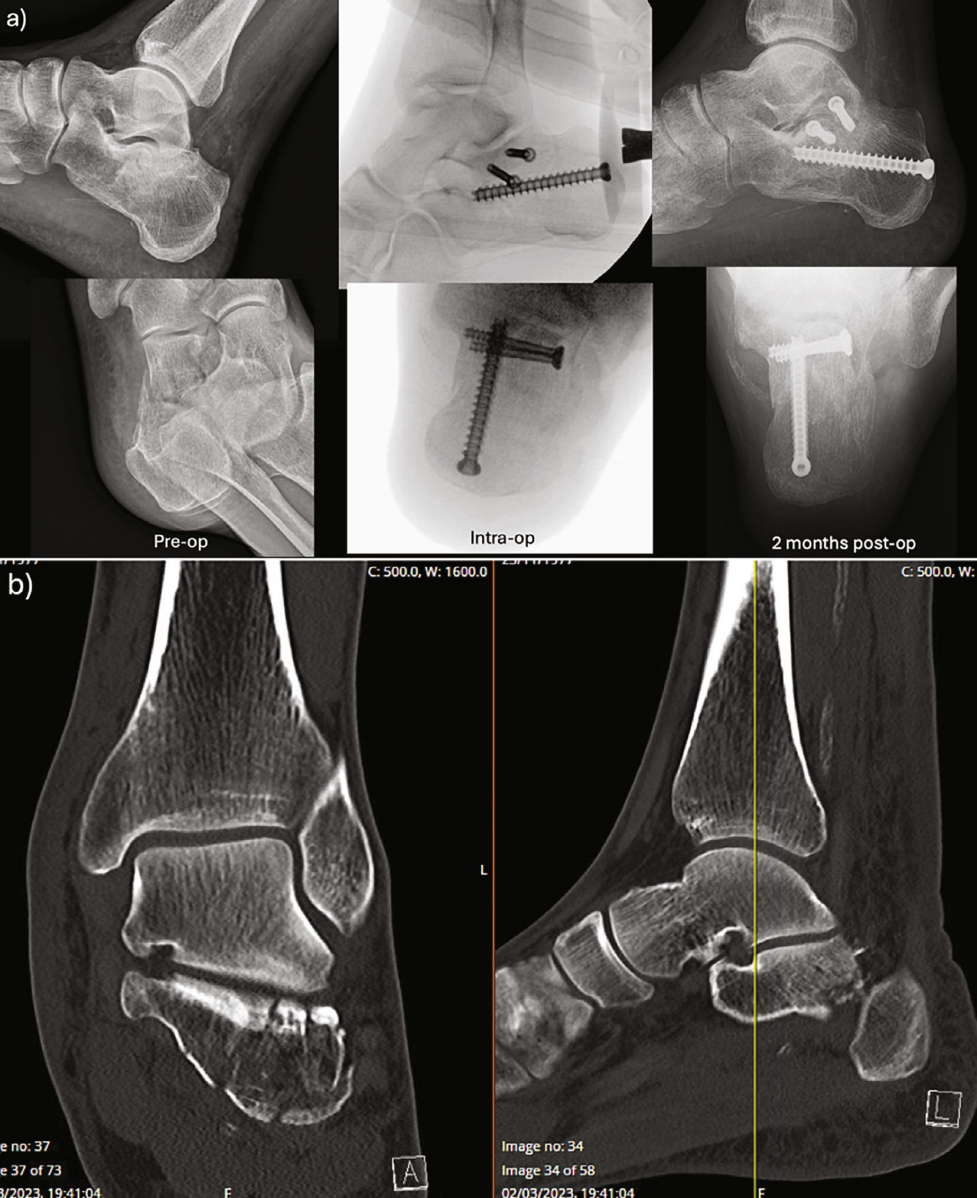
Case B. (a) Pre‐, intra‐ and postoperative x‐rays. (b) Preoperative CT scan of a closed, left‐sided Sanders 3AB calcaneus fracture.
